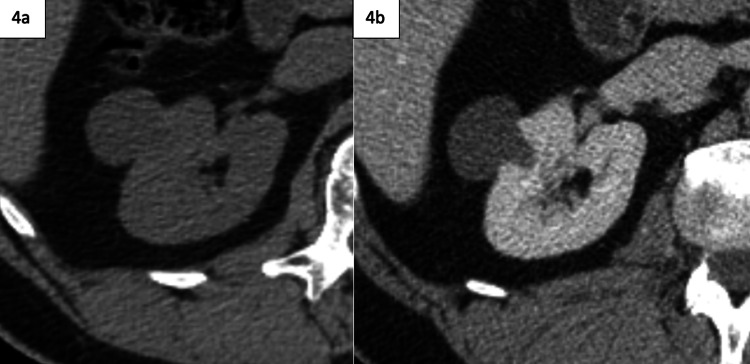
Initial unenhanced CT with follow-up contrast-enhanced CT of renal mass 4a: Unenhanced CT demonstrating a single, exophytic cystic renal lesion measuring less than 20 Hounsfield units, demonstrating the characteristics of a benign renal cyst, requiring no dedicated follow-up. 4b: Follow-up contrast-enhanced CT demonstrating nonenhancement.
